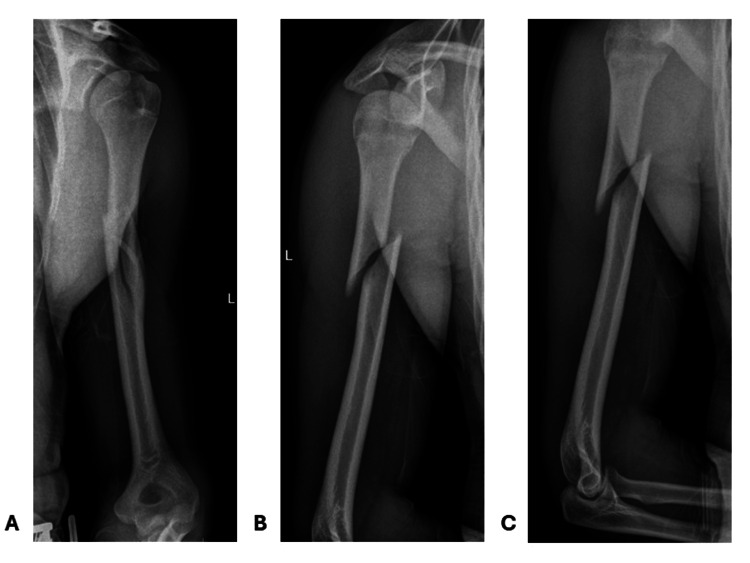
Anteroposterior (A) and lateral (B, C) radiographs from date of injury (10/21/2023)
